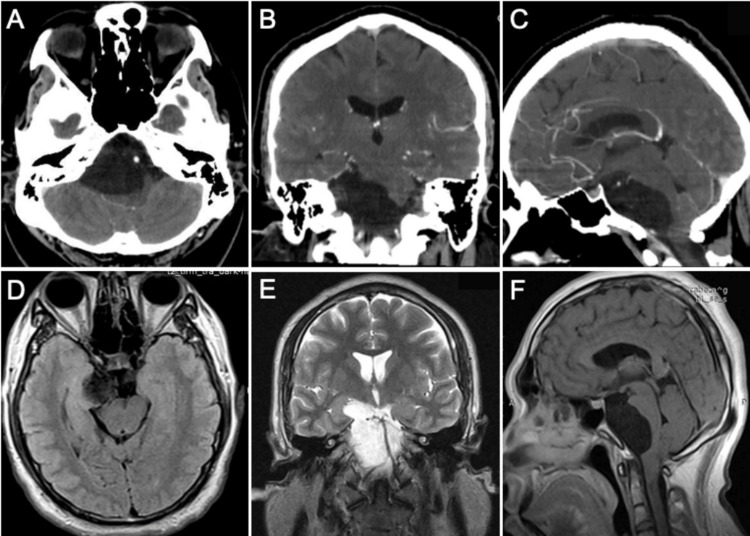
Preoperative MRI. Contrast-enhanced CT showing a cystic lesion displacing the basilar artery to the left side on an axial image (A) and compressing the right uncus on a coronal image (B). The mass effect on the brain stem can be appreciated in the sagittal image (C). The lesion appeared hypointense on T1-weighted MRI (D) and hyperintense on T2-weighted MRI, extending supratentorially, causing compression of the mesial temporal lobe (E). On the sagittal fluid-attenuated inversion recovery (FLAIR) MRI, the cystic content appears heterogeneous with no perilesional edema (F).
